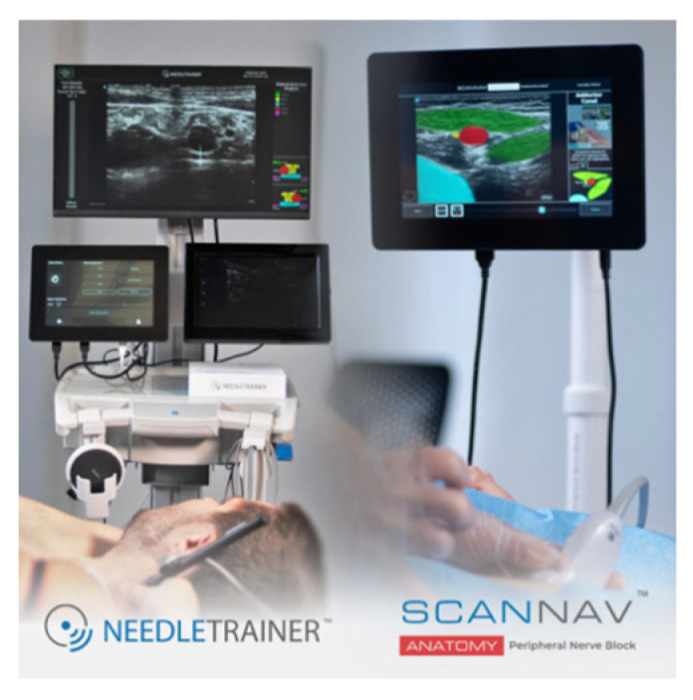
SkanNav Anatomy presenting color-coded anatomy along with an instruction video (from: https://www.intelligentultrasound.com/scannav-anatomy-pnb accessed on 1 January 2024).
